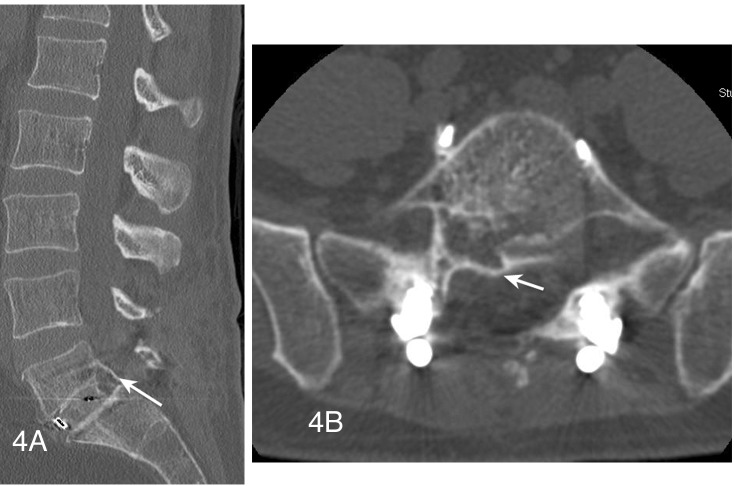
Postoperative sagittal and axial CT images showing calcification along former cyst wall (Figure 4A) Sagittal CT reconstruction image demonstrating a solid fusion across the disc space with calcification posteriorly along the previously seen cyst wall (arrow). (Figure 4B) This also visualized on an axial CT image (arrow) and appears to extend into an area of osteolysis along the posterior inferior vertebrae.
